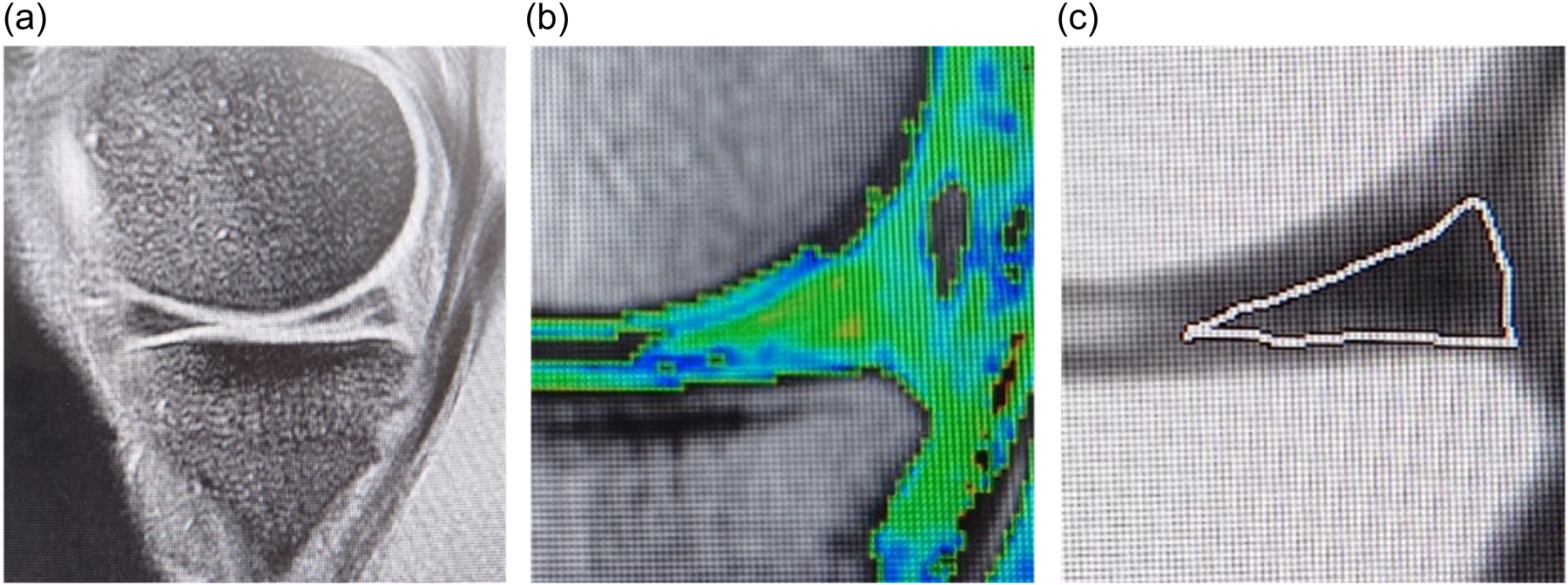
Region of interest for T2 mapping of meniscus tears. (a) Overview of the medial meniscus in the sagittal plane on the T2* sequence. (b, c) Magnification of the white square shown in (a). (b) T2 colour mapping. The warm‐coloured region on T2 colour mapping that is coincident with the region of signal change at the meniscal injury on conventional magnetic resonance imaging was defined as the region of interest for this patient. (c) The area surrounded by the line indicates the whole meniscus. T2 relaxation times were measured on T2 mapping.
